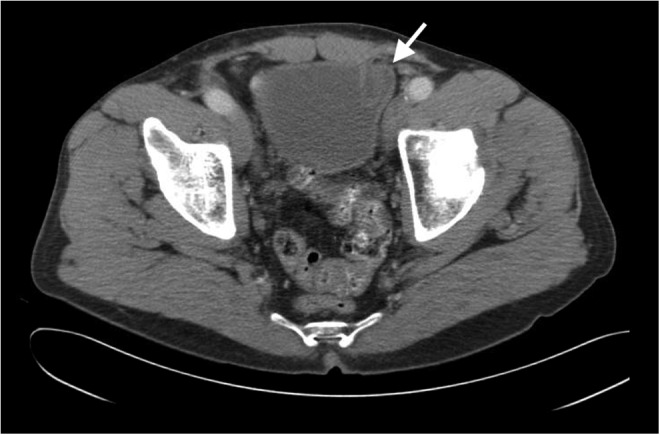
Second image of CT abdomen showing maximal extent of bladder lesion indicated by *white arrow*.
